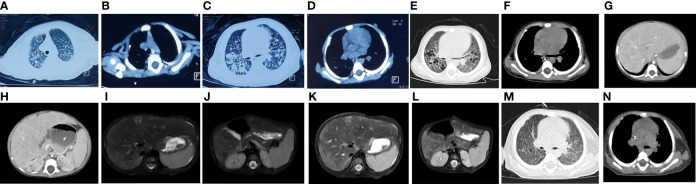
Imaging results for the patient. CT showed that the transmittance of both lungs decreased, and there were many high-density nodular lesions with clear boundaries and cavities in both lungs (A–D) and enhanced CT scan of the chest and abdomen showed thymic dysplasia, diffuse pulmonary nodules (E, F) and liver nodules (G, H). Abdominal MRI showed many short T2 signal sites in (I, J) liver and kidney (K, L). The original bilateral lung fields were absorbed after treatment (M, N).
